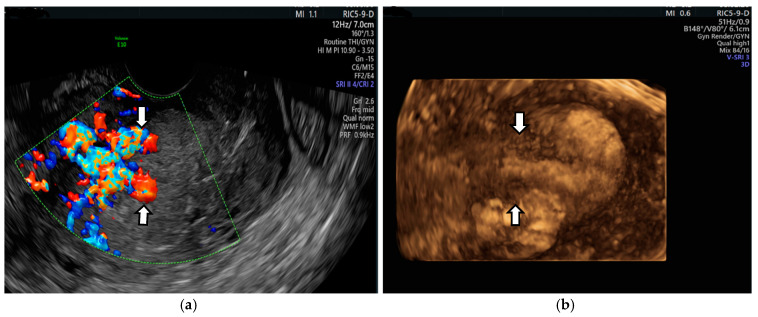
Vaginal ultrasound image of the uterus 3 days after curettage. Arrows show (a) vascular pedicles in the uterine cavity—color Doppler; (b) the same image in three-dimensional ultrasound.
